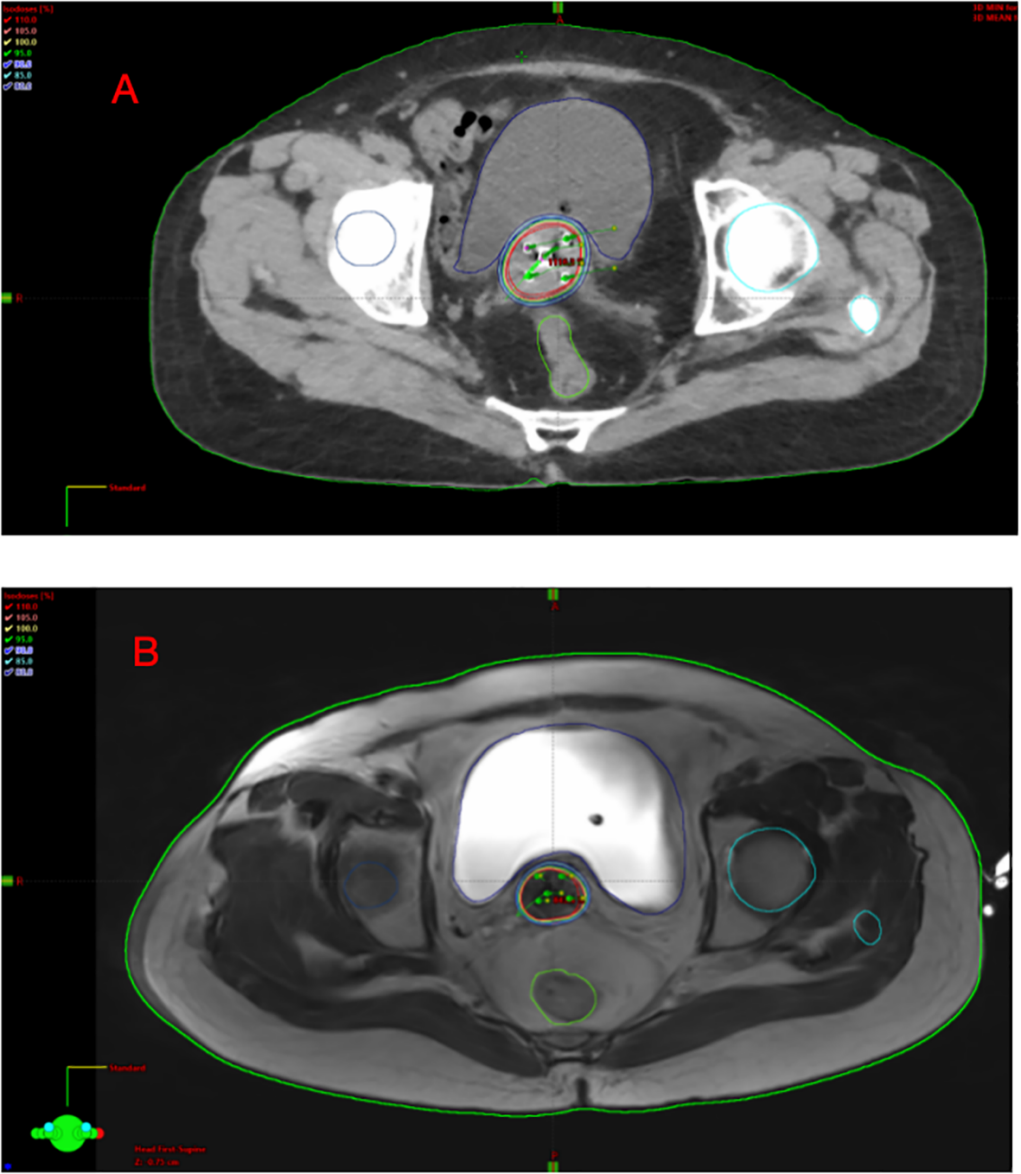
The iso dose of CT scan (A) and iso dose of MRI scan (B).
